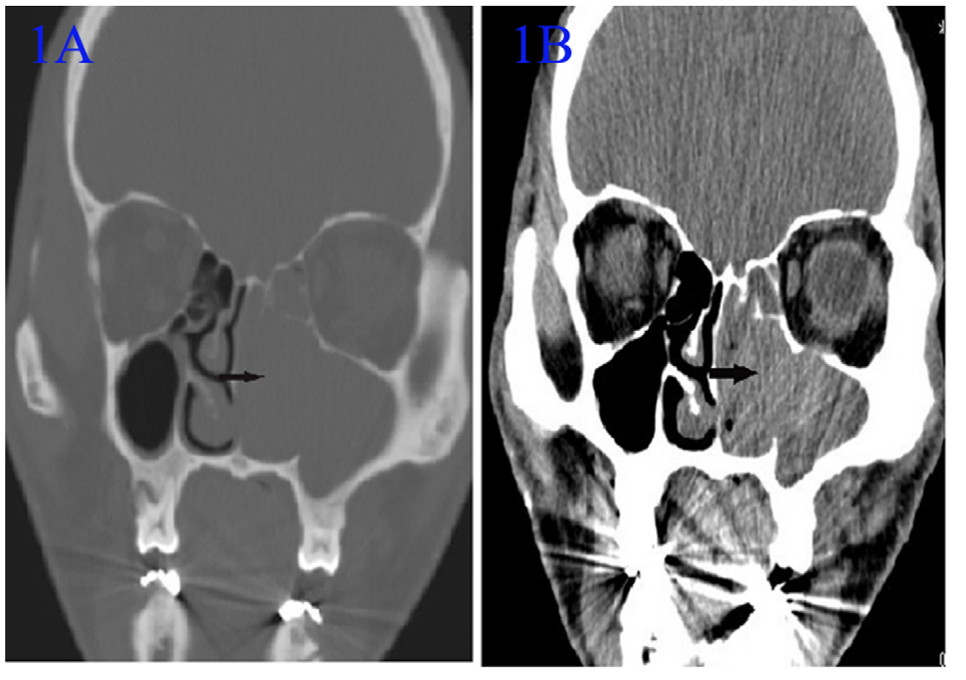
Computed tomography (CT) of a recurrent angiomatous polyp (ANP) in the left maxillary sinus 10 years after ANP removal surgery. CT showed an irregular, soft-tissue mass in the left maxillary sinus. The density was heterogeneous, and the CT value was approximately 45 HU. The medial maxillary sinus wall was completely destroyed (arrow). The mass involved the left nasal cavity and the left ethmoid sinus. A: noncontrast coronal CT in bone window; B: noncontrast coronal CT in soft-tissue window.
